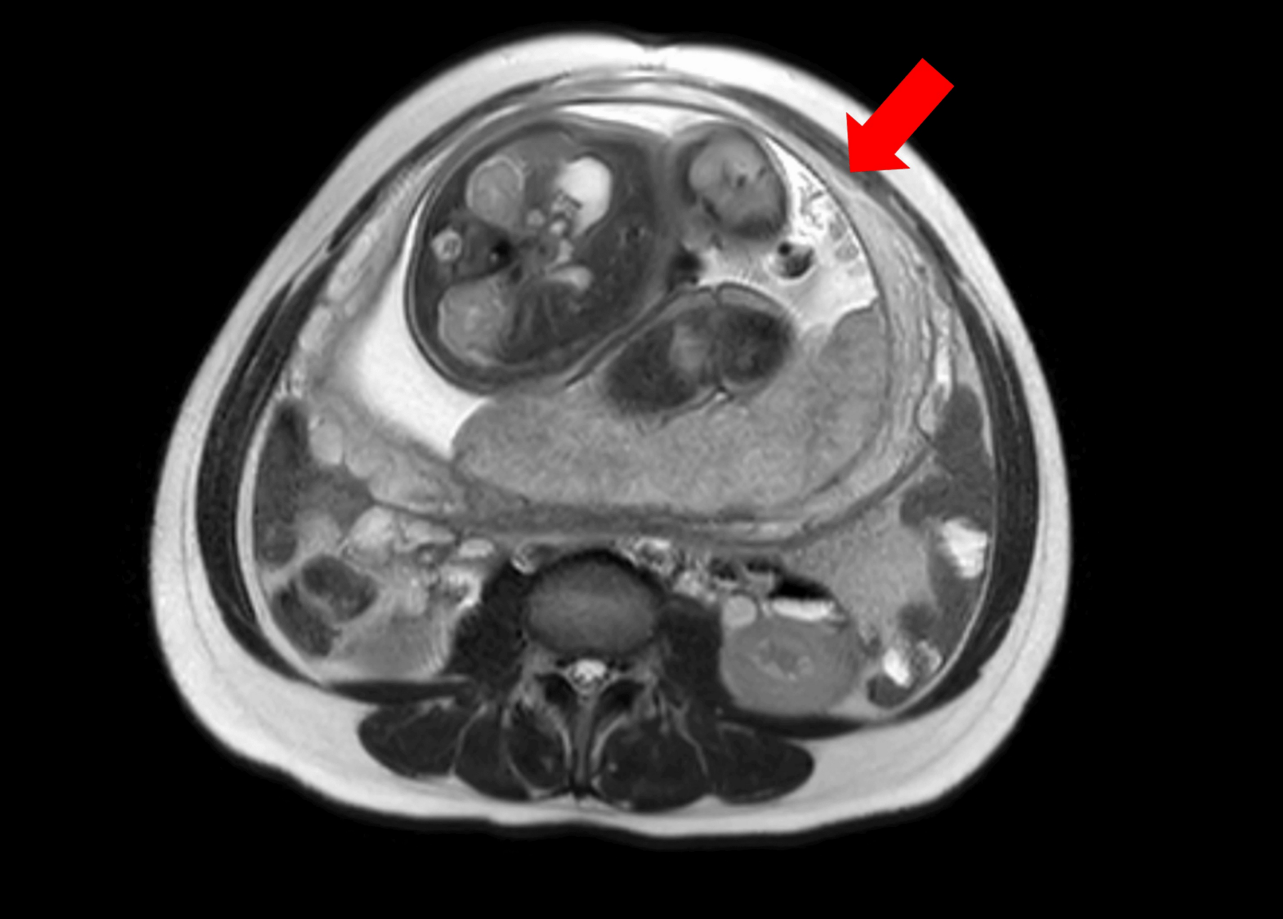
Pelvic MRI examination during pregnancy MRI images during pregnancy suggested no muscle thinning on the left side of the anterior wall (red arrow). There was no obvious thinning of the myoma suggestive of uterine rupture, so delivery at preterm was considered unnecessary.
